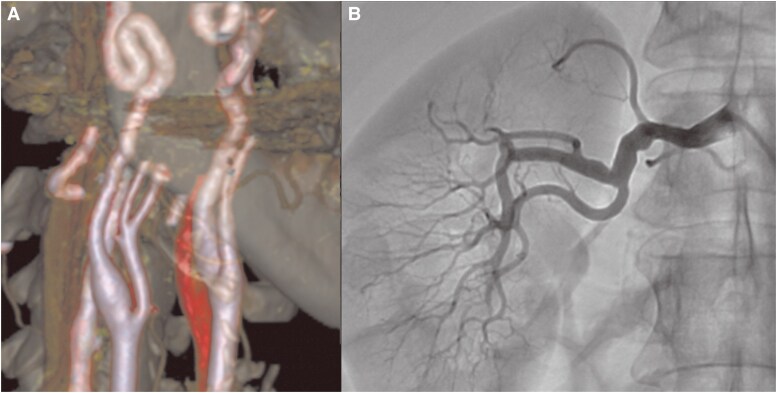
Computed tomography reconstruction of supra-aortic trunks with fibro-muscular dysplasia of internal carotids (*A*); right renal artery angiography with signs of fibro-muscular dysplasia (*B*).
